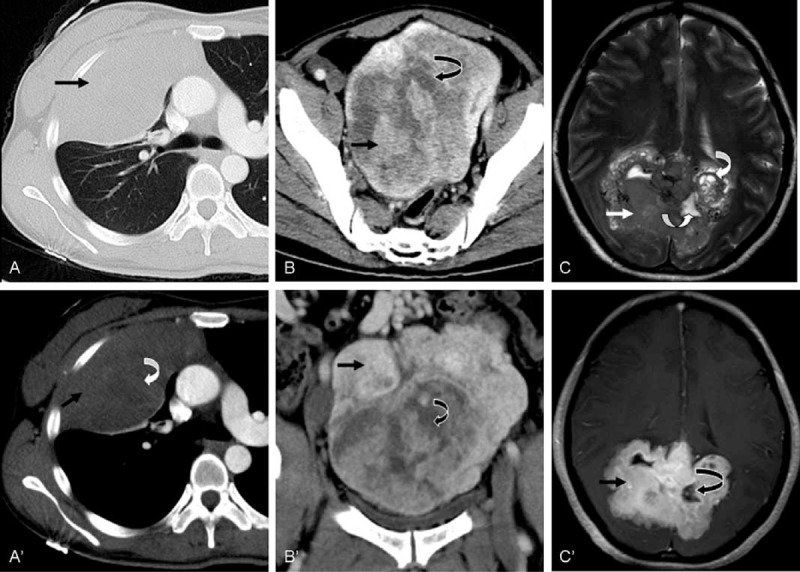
Typical features of larger SFTs. A, A’: A 35-year-old man with an incidental finding of SFT. Parenchymal (A) and mediastinal (A’) contrast material-enhanced CT images showed a round solitary fibrous tumor of the pleura. B, B’: A 54-year-old male presented with a pelvic SFT. Contrast-enhanced CT image (B, axial) and (B’ coronal) views reveal an oval, well-defined heterogeneous mass with patchy hyperdense (tumor tissue, arrow) and hypodense (necrotic tissue, curved arrow) areas and marked heterogeneous enhancement. C, C’: A 41-year-old female patient with a brain SFT. MR T2-weighted images revealed a heterogeneous hypo- and hyperintense mass in the occipital lobe (C). Contrast-enhanced T1-weighted images showed heterogeneous enhancement of the lesion (C’). CT = computed tomography, MR = magnetic resonance, SFTs = solitary fibrous tumors.
